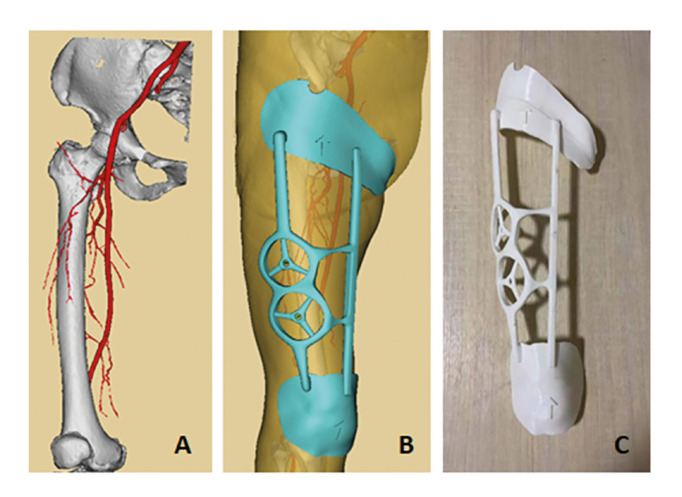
Preoperative digital design and 3D-printing fabrication of fixed positioning guide. A. osseous tissue and perforator vessels were reconstructed in line with CTA images; B. based on the previous results of reconstruction, the 3D printing template was designed; C. The 3D-printing fabrication of fixed positioning guide was completely shaped up.
